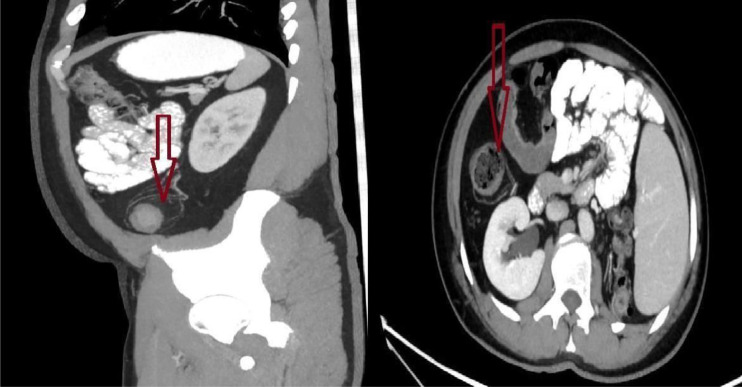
Creeping fat appearances in the sagittal and axial sections of computed tomography.
